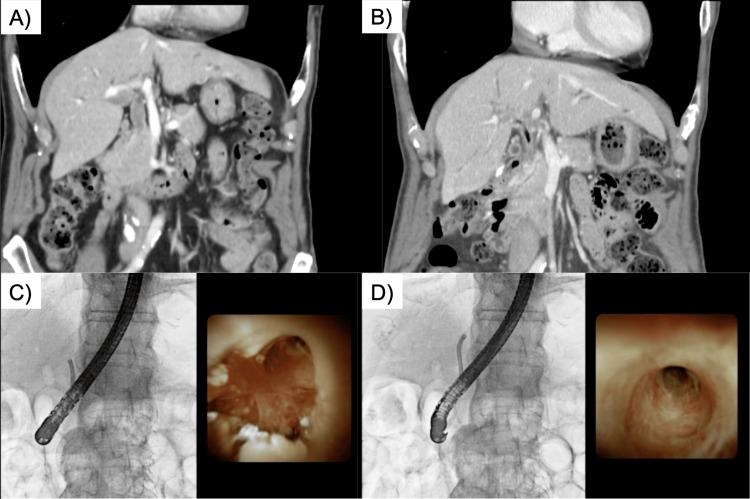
Comparison of CT and oral cholangioscopic images pre- and post-high-dose-rate brachytherapy A) Represents the pretreatment coronal image of contrast-enhanced computed tomography (CT), while B) represents the post-treatment coronal image of contrast-enhanced CT. The solid component in the bile duct that was showing a contrast effect before treatment is obscured. C) and D) represent the post-treatment evaluation images obtained using peroral cholangioscopy (POCS). C) focuses on the hepatic hilum, while D) captures the left bile duct. The papillary lesion observed prior to treatment demonstrates improvement. A biopsy was performed on the suspicious area suggestive of residual disease, revealing evidence of inflammation.
